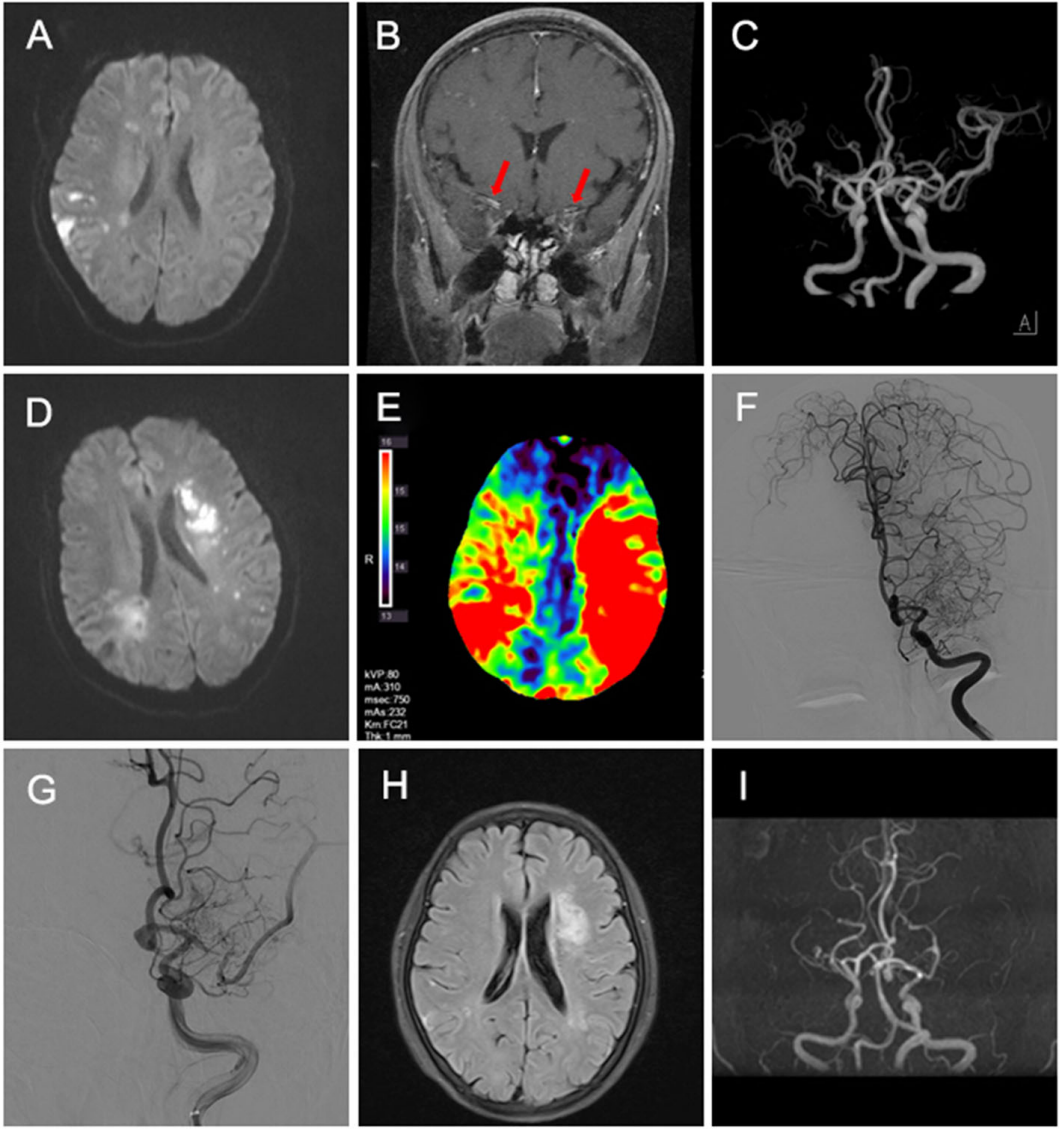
Hypereosinophilia with massive cerebral infarction and middle cerebral artery (MCA) occlusion in a 55-year-old woman. Onset Stage: a Diffusion-weighted magnetic resonance imaging (DWI) revealed multiple acute infarcts in right hemisphere. b Coronal enhanced MRI revealed bilateral middle cerebral artery wall thickening and enhancement (*red arrows*). c MRA showed slightly stenosis in horizontal segment of right MCA. Progression Stage: d DWI revealed new cerebral infarction in left hemisphere. e CTP showed a significantly longer peak time (TTP) in both hemispheres. f DSA showed M1 segment of left MCA occlusion. After endovascular therapy, partial MCA recanalization g. Recovery Stage: Brain flair MRI h demonstrated focal ischemia changes. i Follow-up brain MRA showed bilateral middle artery branches occlusion
